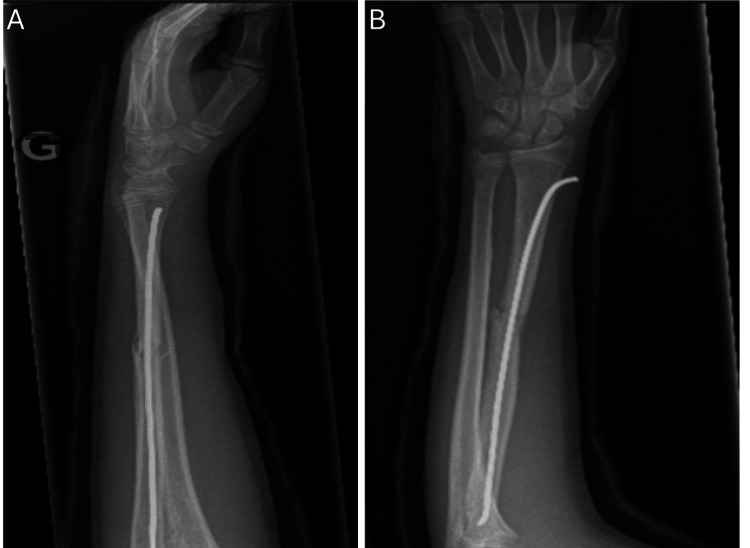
Four-week radiograph showing a mid-diaphyseal radial fracture undergoing consolidation, with pin osteosynthesis and anatomical realignment A: Lateral view B: Anteroposterior view
